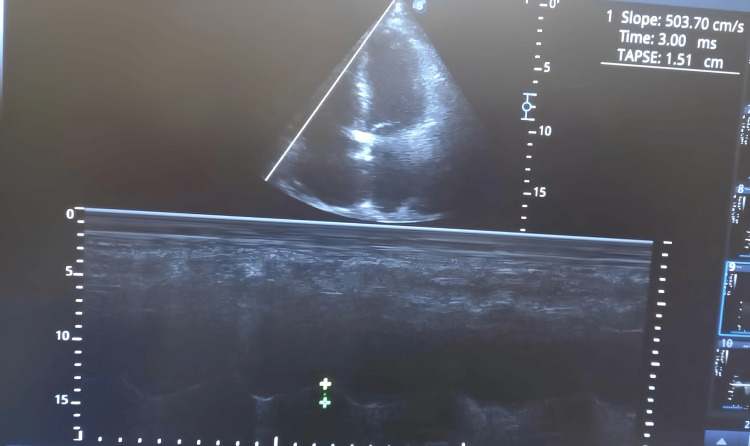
M-mode echocardiographic measurement of Tricuspid Annular Plane Systolic Excursion (TAPSE) from the apical four-chamber view Tricuspid Annular Plane Systolic Excursion (TAPSE), mm: millimeter, ms: millisecond.
